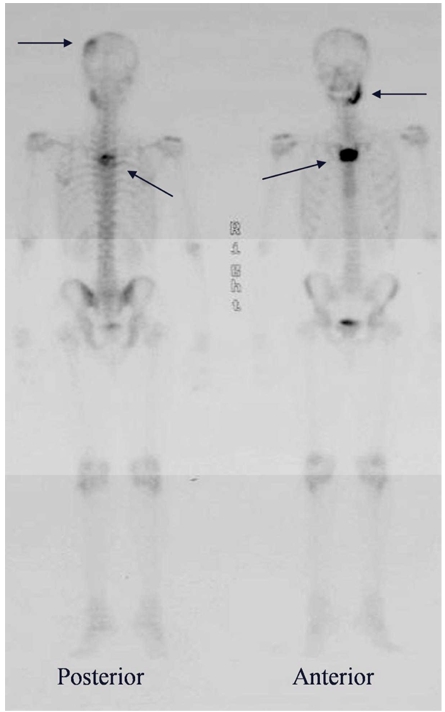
Radionuclide bone scintigraphy using MDP 99m-Technicium showing intense radiotracer uptake in the left side of mandible, manubrium sterni, upper thoracic vertebra and the skull vault (arrows).
